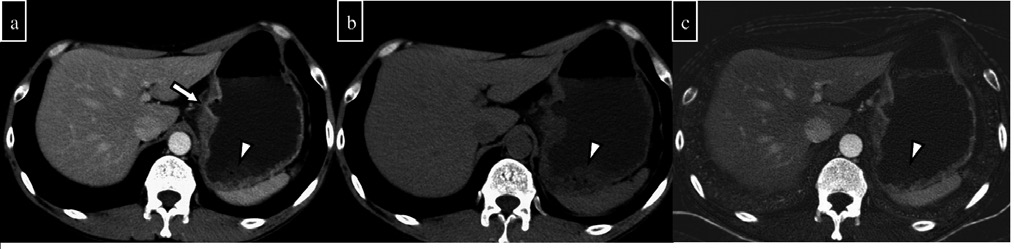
A 52-year-old man has a history of gastric ulcer bleeding. Owing to haematogenous vomiting, single-phase CT was performed. (a) Conventional CT shows an ulcer in the gastric lesser curvature (arrow). Gastric contents show slightly high density on conventional CT (arrowheads). (b) VNC shows a slightly high density of gastric contents (arrowheads). (c) The iodine map shows no apparent contrast agent content in the gastric contents. Haematoma is suspected rather than active bleeding. No obvious bleeding was observed on the endoscopy. VNC, virtual non-contrast CT.
